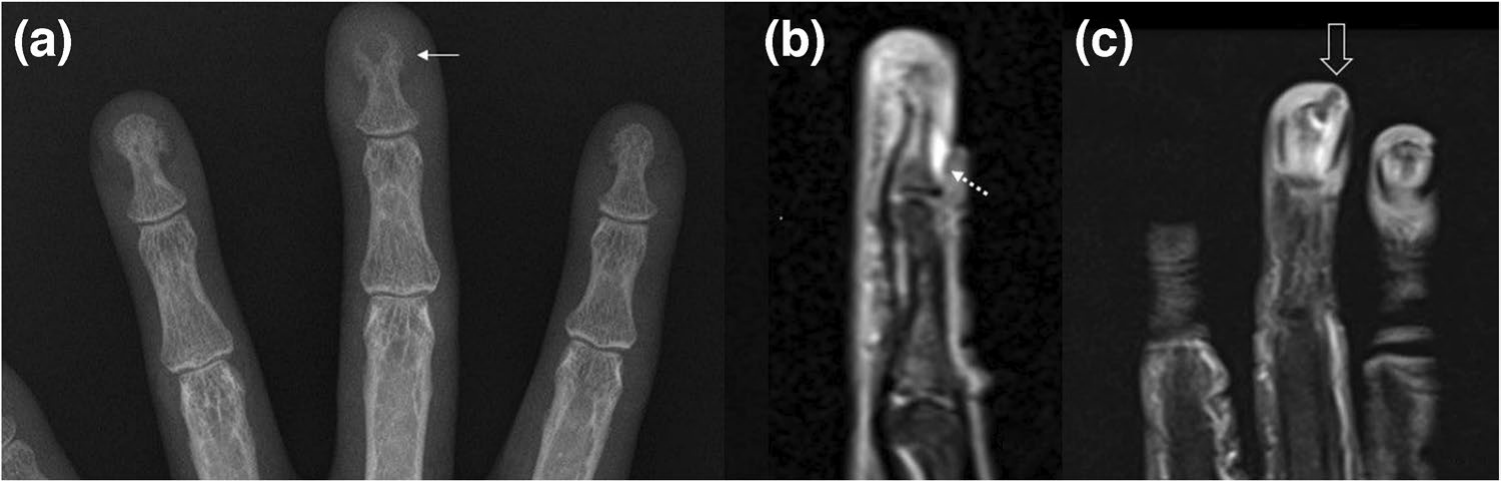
Anteroposterior radiograph (a) and sagittal fat-suppressed proton density-weighted (fs-PDW) image (b) of a 52-year-old woman with paronychia and associated osteomyelitis of the distal phalanx are shown. Note the lytic bone lesion at the tuft of 3rd distal phalanx (arrow in a) and small fluid collection in the fs-PDW image (dashed arrow) underneath the skin fold. (c) Coronal postcontrast fs-T1W image depicts contrast enhancement along with a micrometallic artifact (open-arrow) possibly from a previous penetrating metallic foreign body trauma, although the patient could not recall a specific history
